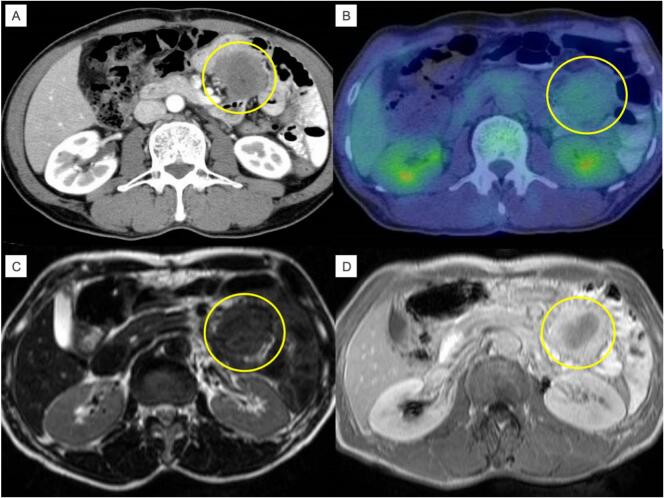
Preoperative image. CT showed a soft-tissue tumor in the mesentery of the jejunum (yellow area) (a). PET-CT showed the presence of an area with pale accumulation of FDG (b). T2-weighted MRI showed a low signal (c). T1-weighted MRI showed a low signal (d). (For interpretation of the references to colour in this figure legend, the reader is referred to the web version of this article.)
